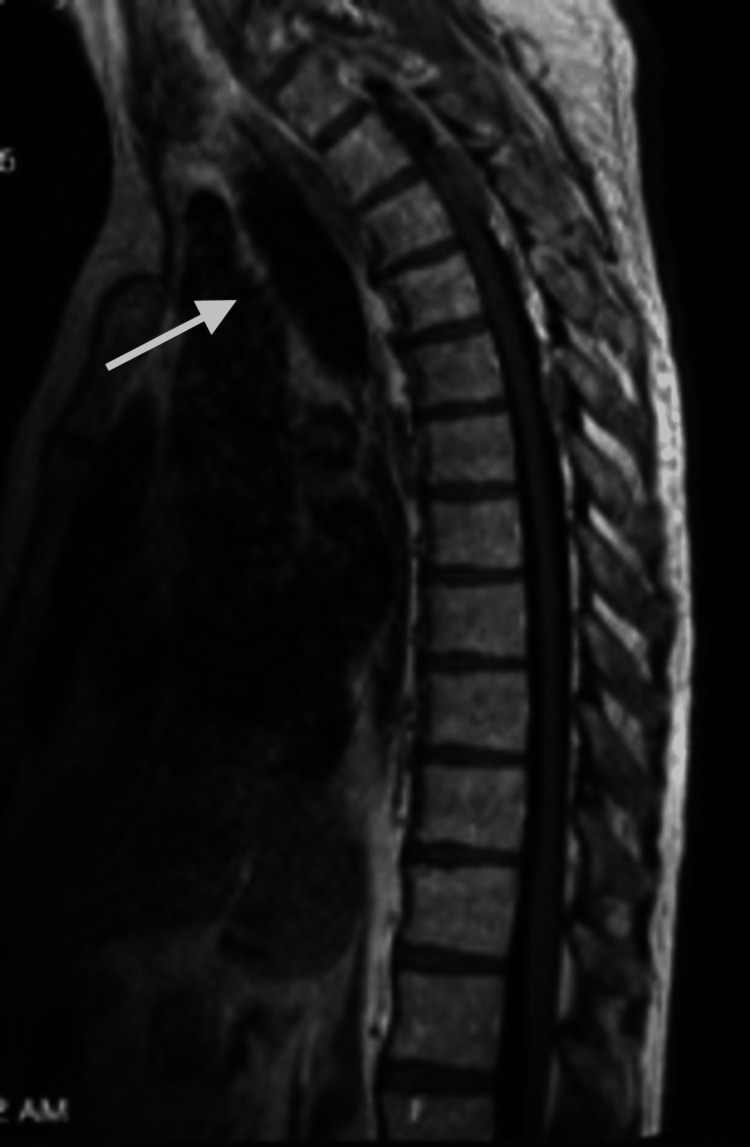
MRI thoracic spine w/wo contrast showing an epidural hematoma extending along the posterior aspect of the thoracic spine from T2-T6, left greater than right and most prominently at the level of T3 where there is mild mass effect over the left posterior spinal cord (white arrow)
